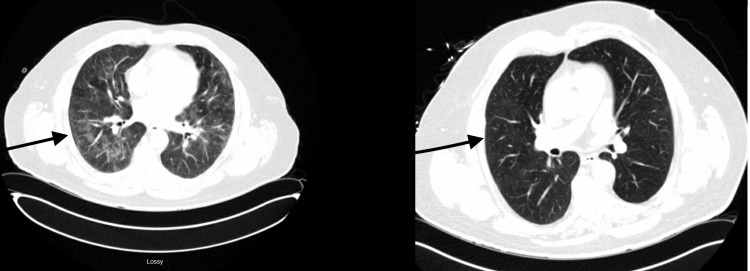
CT Chest Admission CT chest (left) shows bilateral alveolar infiltrates felt to represent viral pneumonia. Six weeks follow-up CT chest (right) shows significant improvement in the previously described bilateral alveolar infiltrates.
